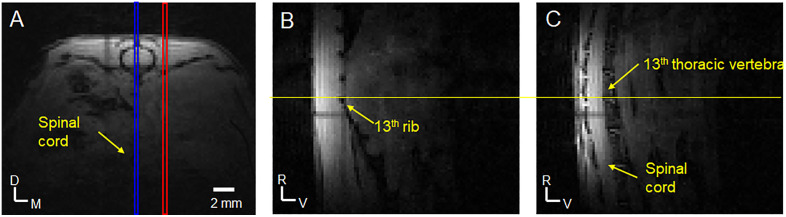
Anatomical images to identify vertebral levels. Based on the axial image (A), two sagittal images (B & C) were acquired as indicated by red box and blue box in (A). Image (B) was used to identify the position of 13^th^ rib (the lowest circular region with hypo-intensity in the caudal direction). From this anatomical marker, the 13^th^ thoracic vertebra is identified (C). DRG MRI data in axial slices were acquired around the 13^th^ thoracic vertebra level. D: dorsal; V: ventral; M: medial; R: rostral.
